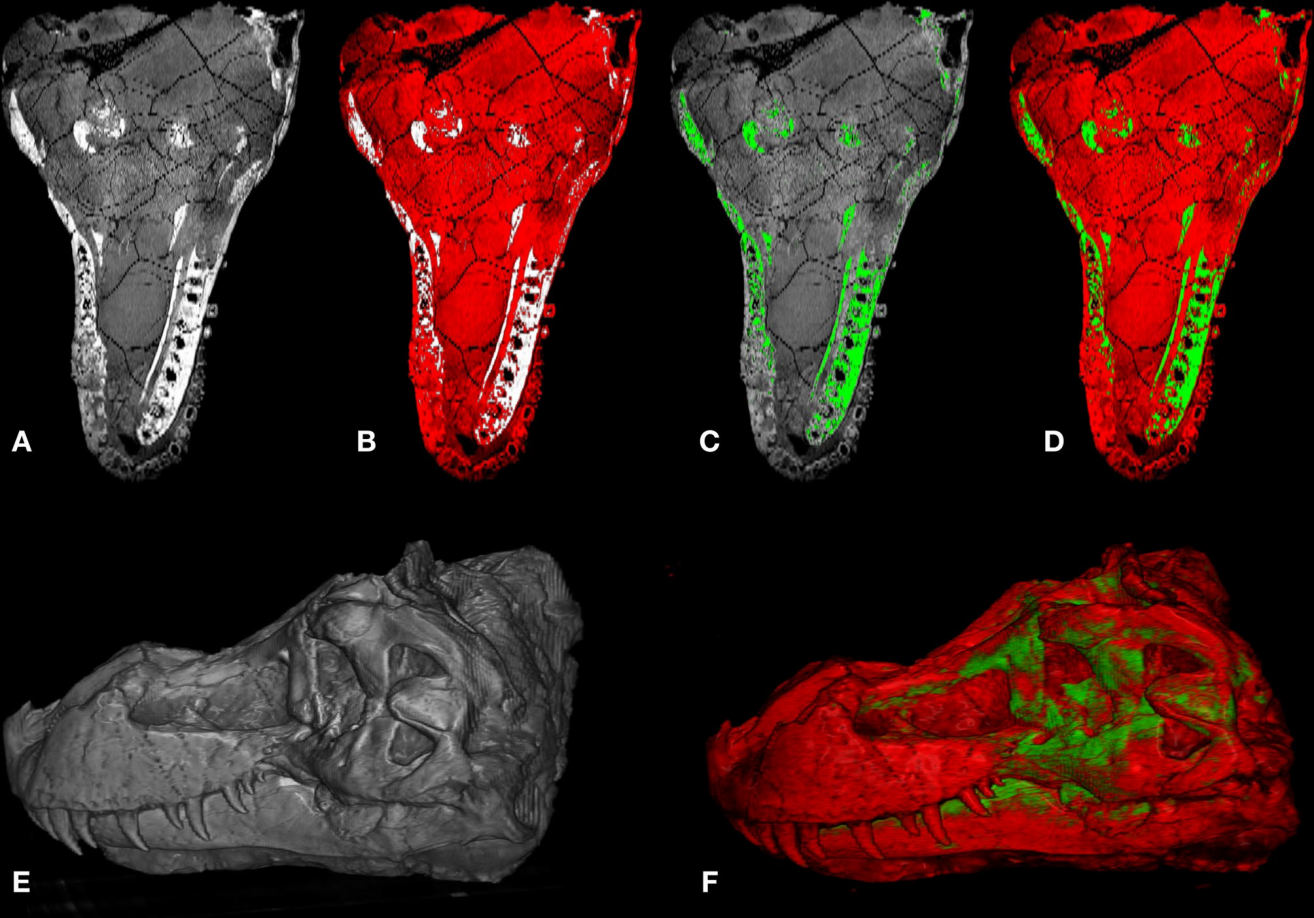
Automated shape analysis and segmentation of Tyrannosaurus Sue skull. (A) Slice through original CT volumetric data; (B) SWD of low intensity values, (C) SWD of high intensity values; (D) combined display of (B, C); (E) unsegmented SWD reconstruction of entire volume, (F) combined segmented SWD volumes.
